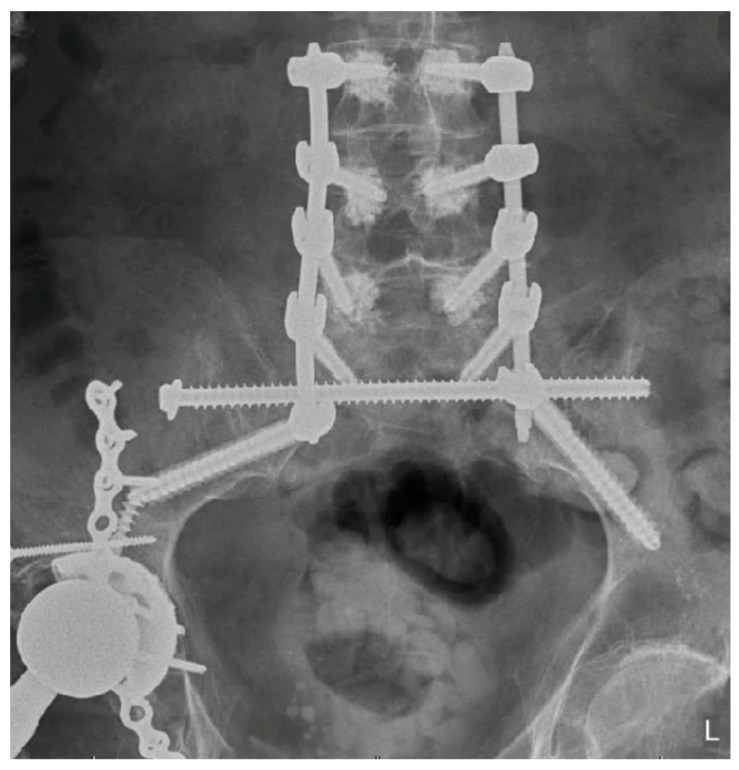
AP Radiograph of cement augmented spinopelvic fusion.
